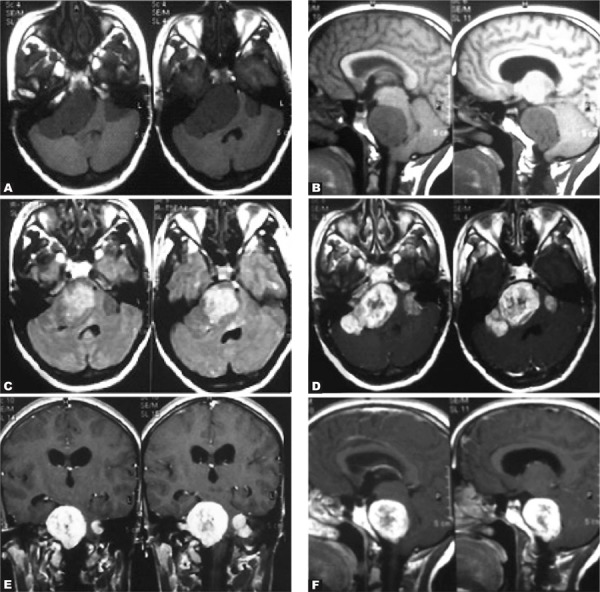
A 23-year-old girl with sensory-neural hearing loss. A and B. Axial and sagittal T1-weighted MR images of the brain. C. Axial FLAIR image. D-F. Axial, coronal and sagittal T1-weighted with contrast MR images.
